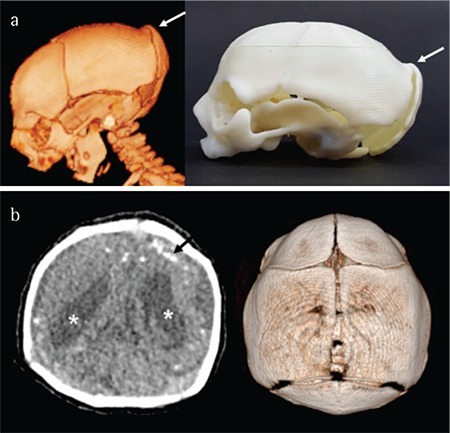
Three-dimensional sagittal reconstruction from computed tomography scan and corresponding three-dimensional printing. The skull has collapsed appearance (arrow) (a), axial plane shows frontal lobe calcifications (arrow), ventricular dilatation (*) with three-dimensional axial reconstruction (b)
